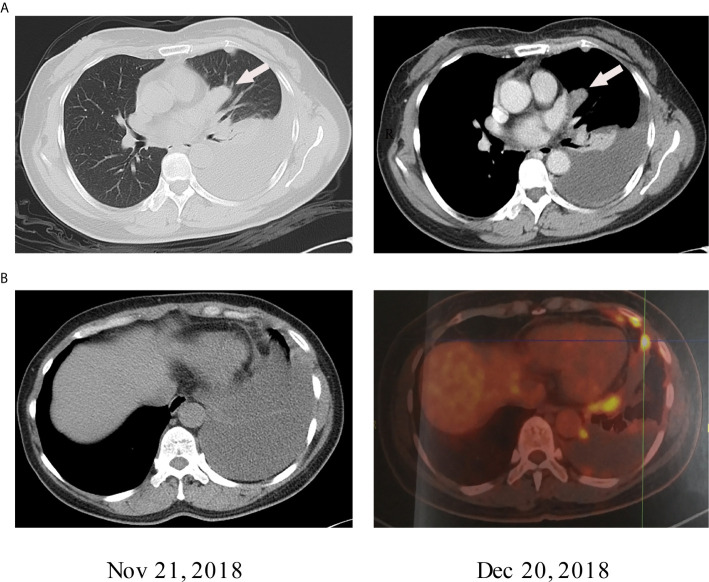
(A) The lung windows show 2.3 cm × 2 cm nodules with uniform density next to the heart margin of the left lung segment. Contrast-enhanced CT of mediastinal windows shows that the nodules were uniformly strengthened. (B) The PET-CT results of the patient on December 20, 2018, showed a decrease in pleural effusion compared to the PET-CT results on November 21, 2018.
